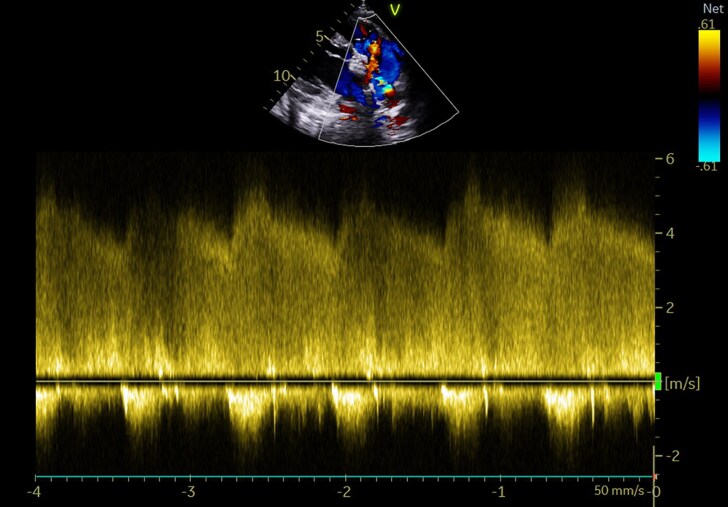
Continuous Doppler echocardiography showing the flow through the patent ductus arteriosus. A continuous flow with a systolic peak of 98 mmHg.
